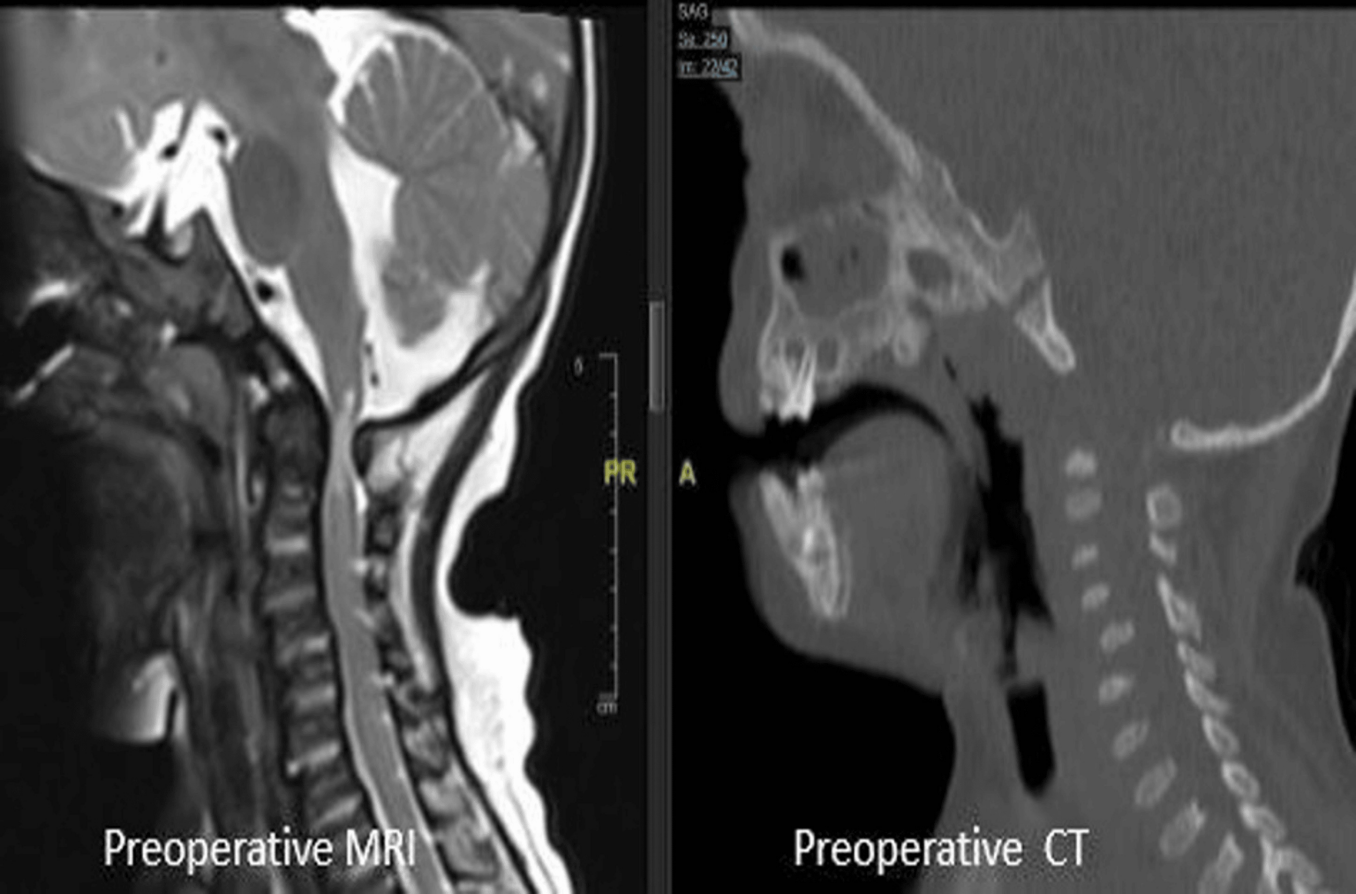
Case 4: Preoperative CT and MRI images of the cervical spine showing severe cervical stenosis.
